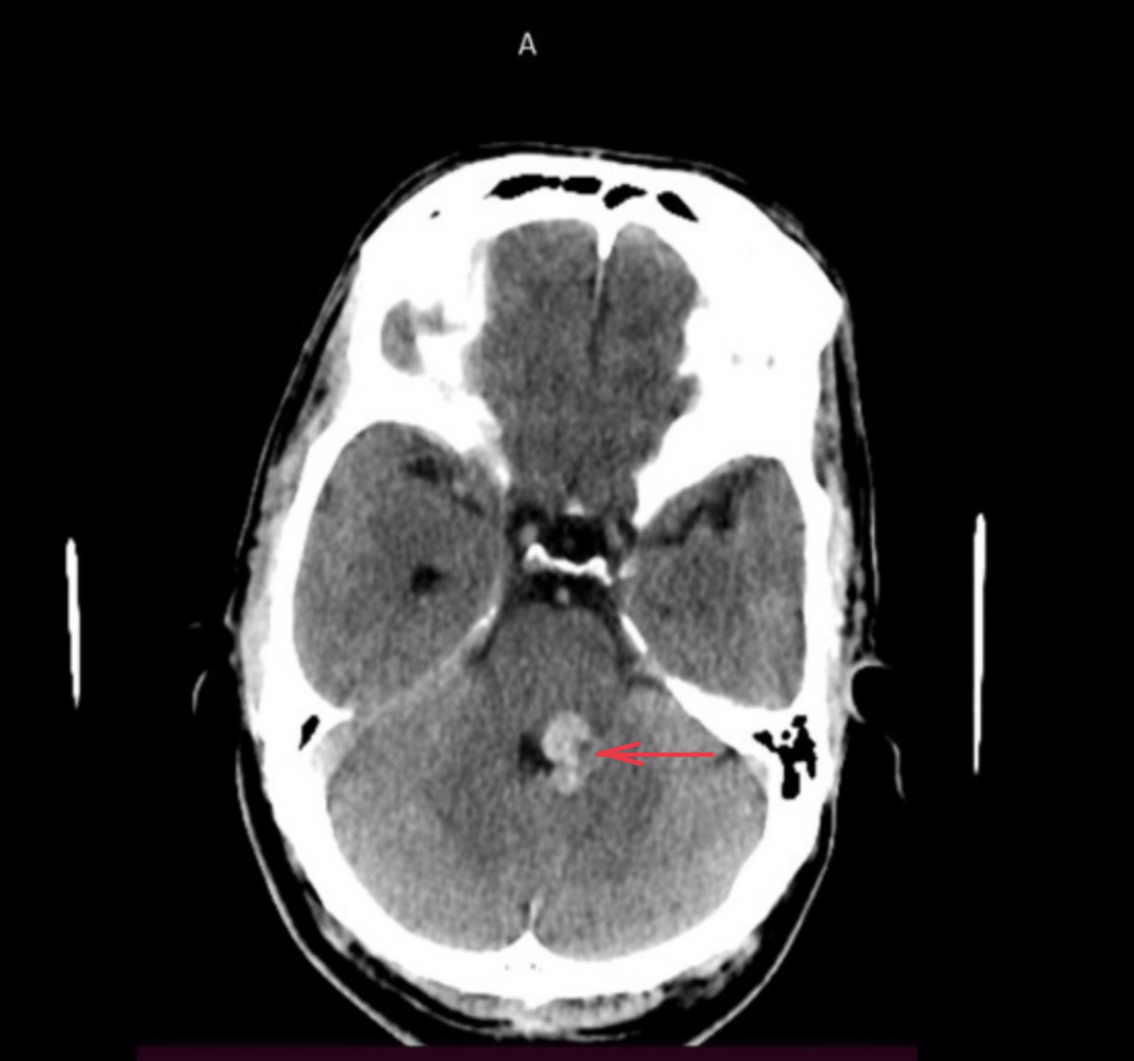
CT brain showing hyperdense lesion involving the left hemipons and left middle cerebellar peduncle indicating a hemorrhage pointed using red arrow CT: Computed tomography
